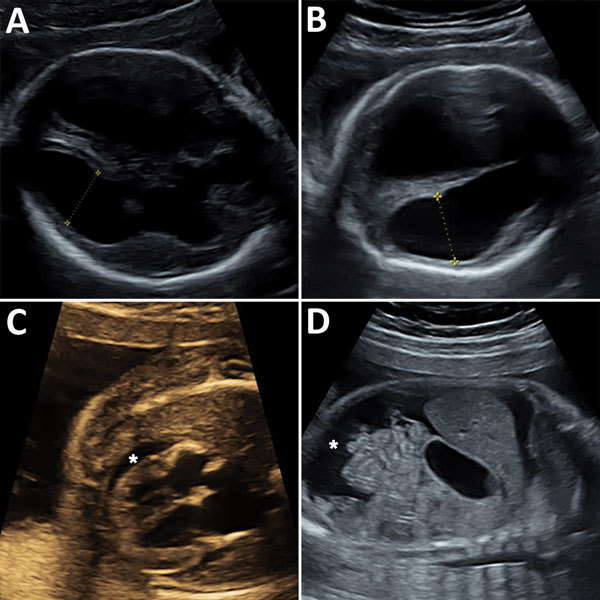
Ultrasonography of congenital microencephaly caused by infection with lymphocytic choriomeningitis virus diagnosed in the fetus of a 29-year-old pregnant women at 23 weeks’ gestation. A) Fetal brain at 23 weeks’ gestation showing symetric ventriculomegaly (14 mm). Yellow symbols indicate axis at which size of cerebral ventricle was measured. B) Fetal brain at 26 weeks’ gestation showing symetric ventriculomegaly (20 mm) and thinning of the cortical mantle. Yellow symbols indicate axis at which size of cerebral ventricle was measured. C) Fetal heart at 24 weeks’ gestation showing pericardial effusion (*) and cardiomyopathy with hyperechogenic muscle. D) Sagittal section of fetal abdomen at 26 weeks’ gestation showing ascites (*).
